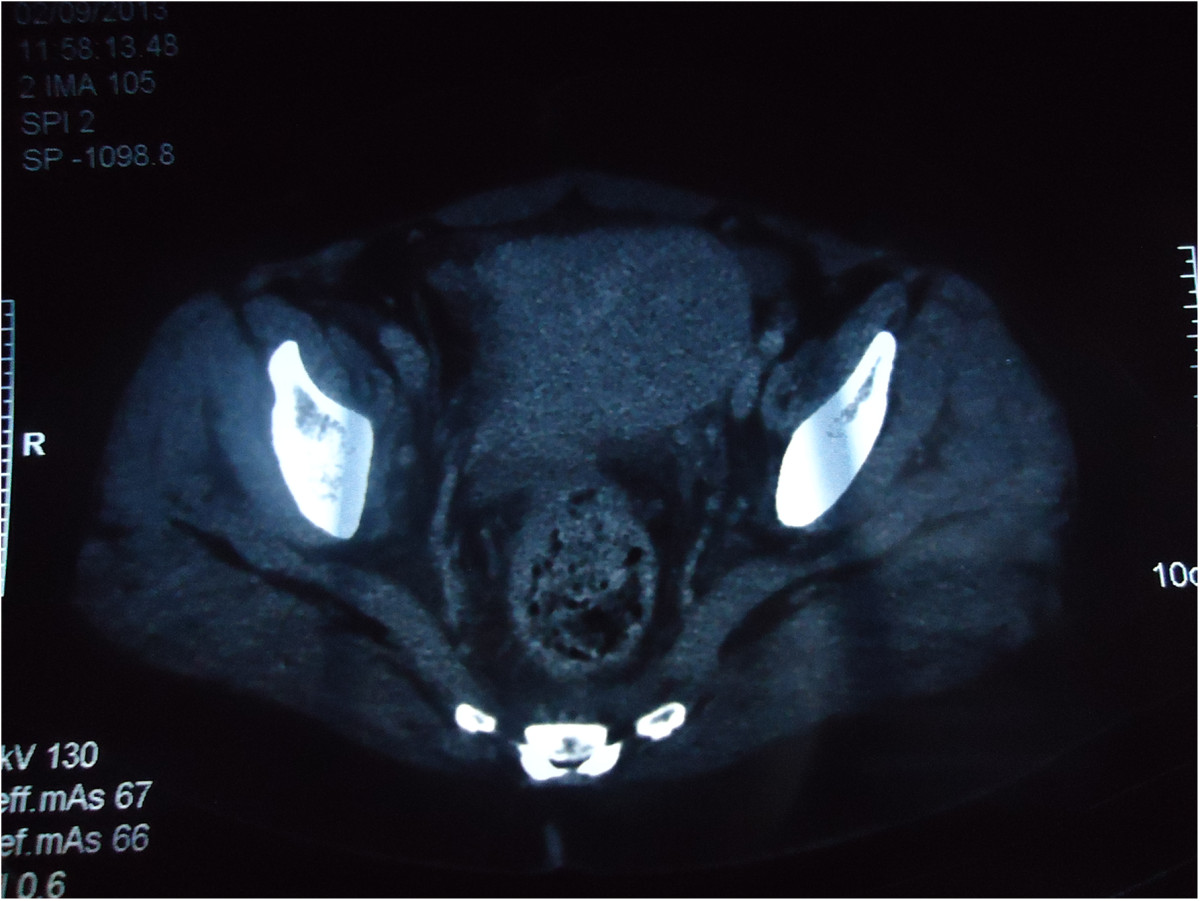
Computed tomography slice of the patient pelvis showing a cervico-isthmic mass.
